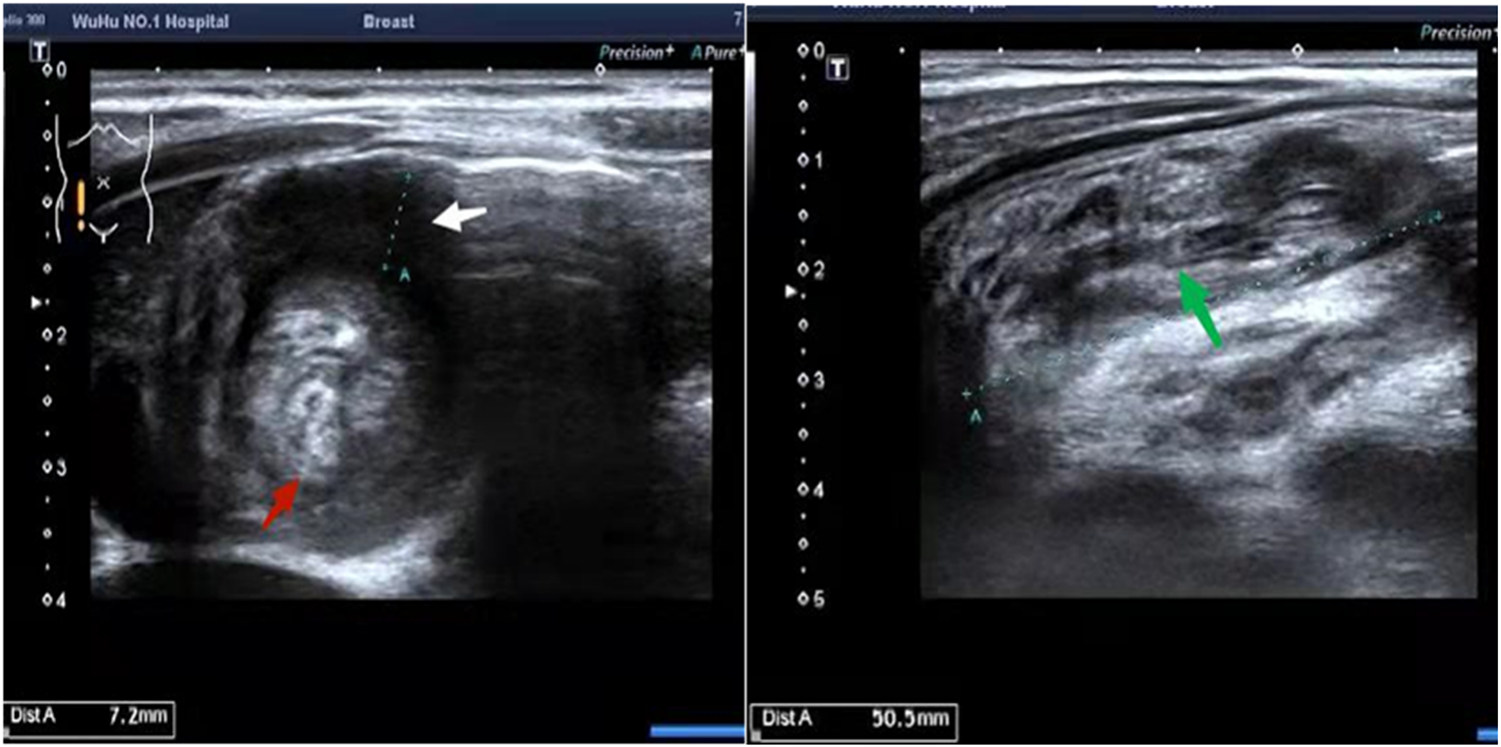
Abdominal ultrasonography reveals the presence of the concentric circle sign in the transverse section (indicated by red and white arrows) and the sleeve sign in the longitudinal section (indicated by a green arrow).
